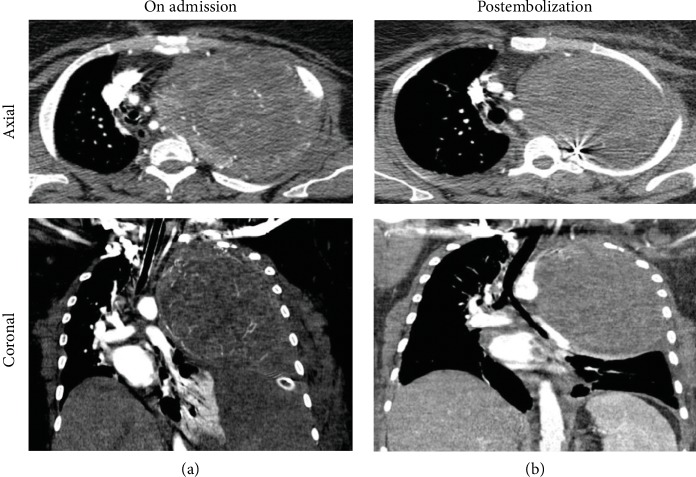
CT angiography of chest: (a) a large 14 × 12 × 8 cm mass in the left upper mid hemithorax with the left lung collapse; (b) markedly reduced vascularization postembolization.
